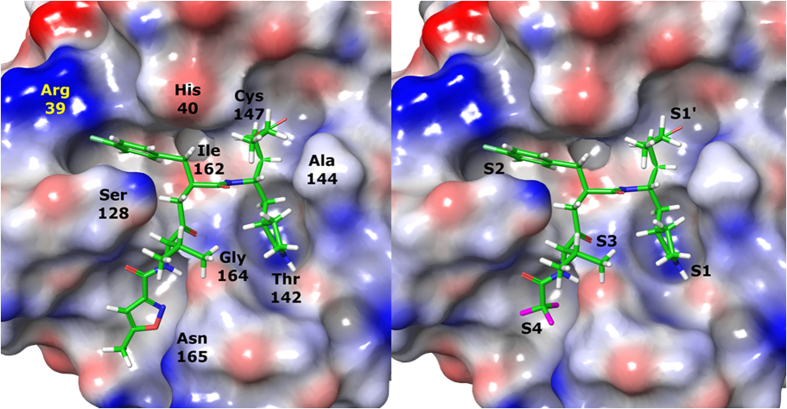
Structures of Rupintrivir and compound 9 at the EV71 3 C protease active site revealed minimal participation of S4 subsite in interactions with both compounds. Rupintrivir-EV71 3 C protease X-ray structure (left) and compound 9 modeled into EV71 (right). The protease is shown with an electrostatic surface and the inhibitor in tube with green carbon, red oxygen, blue nitrogen, magenta fluorine and white hydrogen. Key residues (left) and the binding subsites (right) are labeled.
